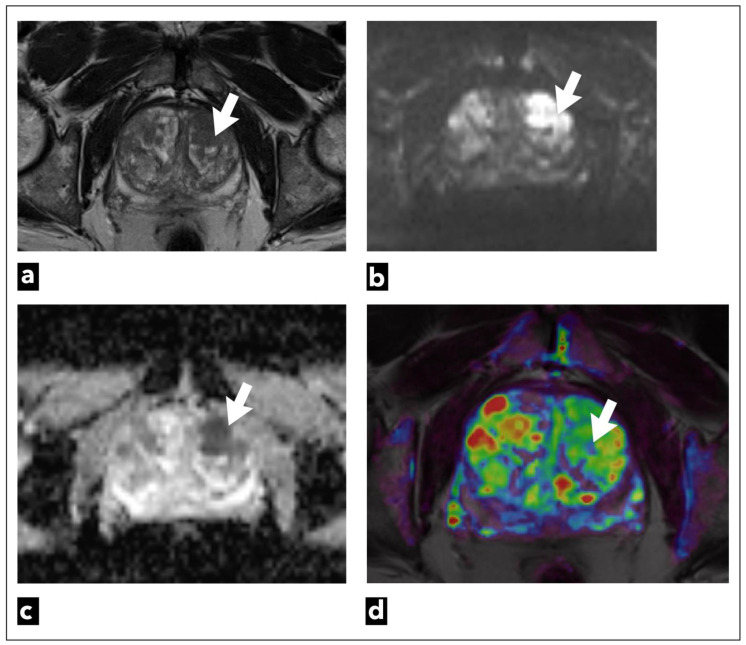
mpMRI shows a left transitional zone prostate lesion (white arrow) in a 69-year-old male patient, which was confirmed to be cancer on biopsy; Gleason score 4 + 3 = 7. It can be seen on (a) T2W as a hypointense focal lesion. The lesion is obscured on (b) DWI b = 1400 s/mm^3^ and (c) ADC maps that are of suboptimal diagnostic quality due to artifacts. DCE-MRI does not demonstrate early contrast enhancement on the (d) pharmacokinetic quantitative colour-coded parametric map for K_trans_.
